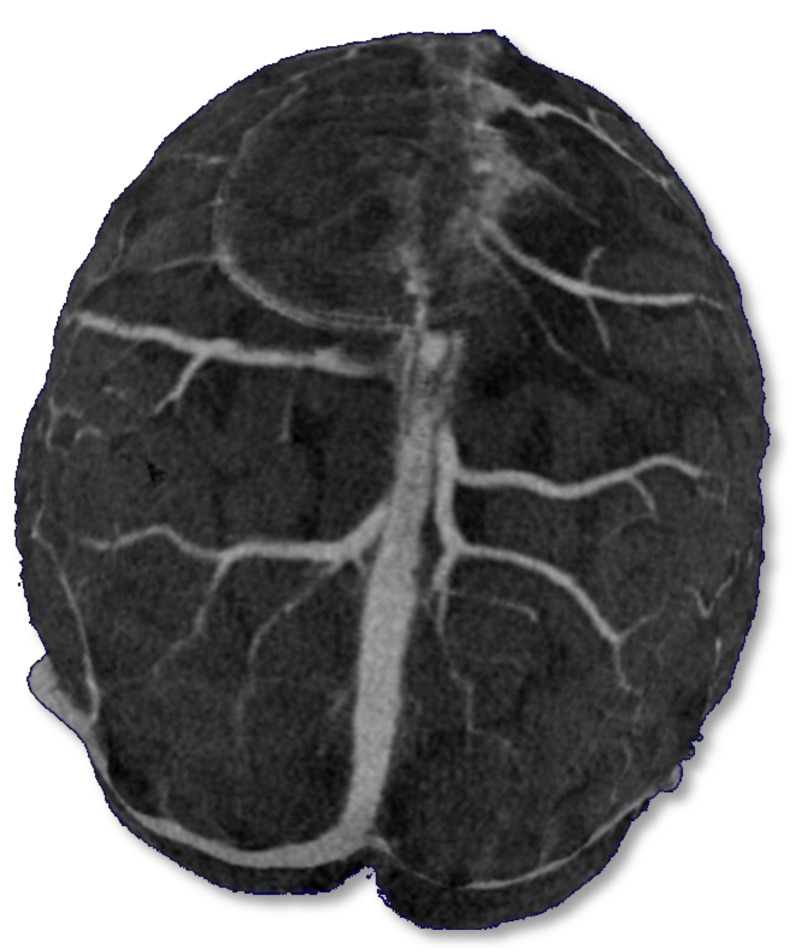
Volume rendering reconstructions of cerebral CT venography. Thrombosis of the anterior part of the superior sagittal sinus.
